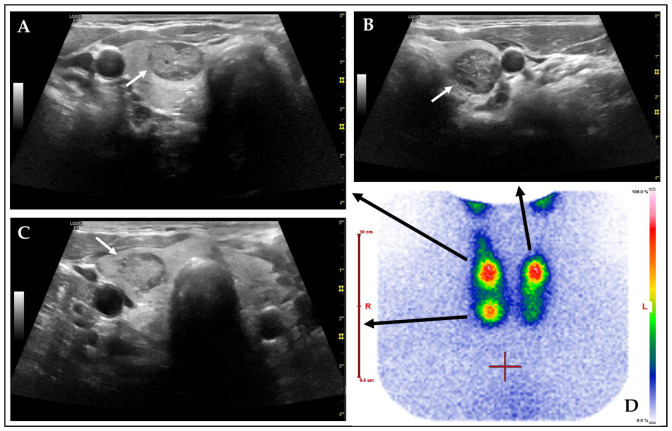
Representative US and Tc-99m-pertechnetate thyroid scintigraphy. Example of a TS with trifocal AFTNs (D). Associated TNs (black arrows) are shown on transverse CL US image captures (A–C, white arrows). (A) and (B) are located in the right lobe, C in the upper left lobe. All AFTNs displayed obtained elevated TIRADS scores with recommendation for ACR FNC. Each of them is exhibiting punctuate echogenic foci.
